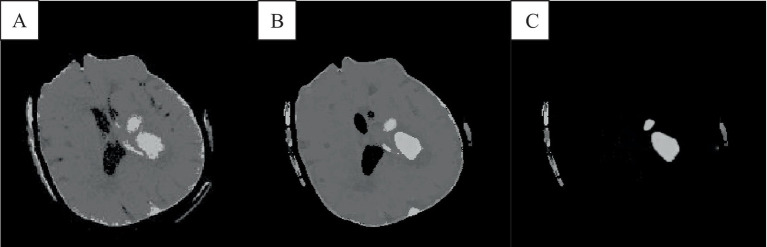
The result of the improved brain CT image clustering operation. A was the result of adding the spatial algorithm, B showed the result of the improved algorithm, and C showed the result of the analysis of the singular point by the improved algorithm.
